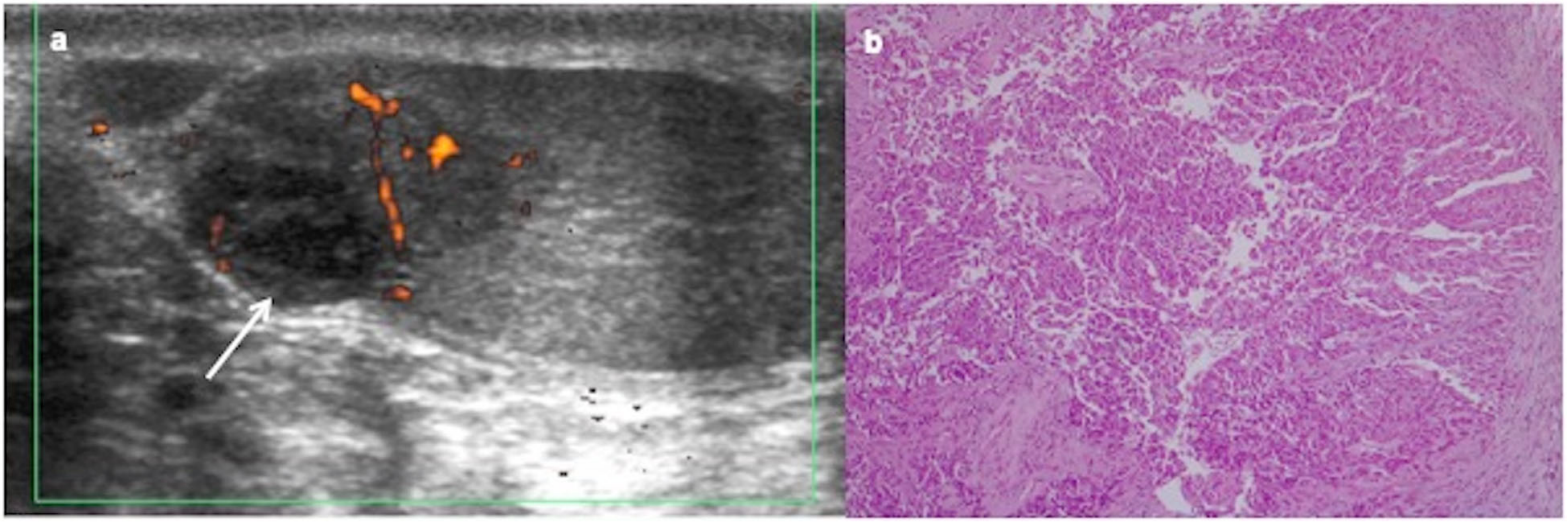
Embryonal carcinoma. A 12-year-old boy with right testicular mass since 2 weeks ago. a Longitudinal ultrasound view of the right testis and colour Doppler show hypoechoic mass with growth invading the tunica albuginea (arrow) and a very slight increased perfusion. A radical inguinal orchiectomy is practised after intraoperative biopsy. b Atypical polymorphic cells with glandular pattern and infiltration tunica albuginea
